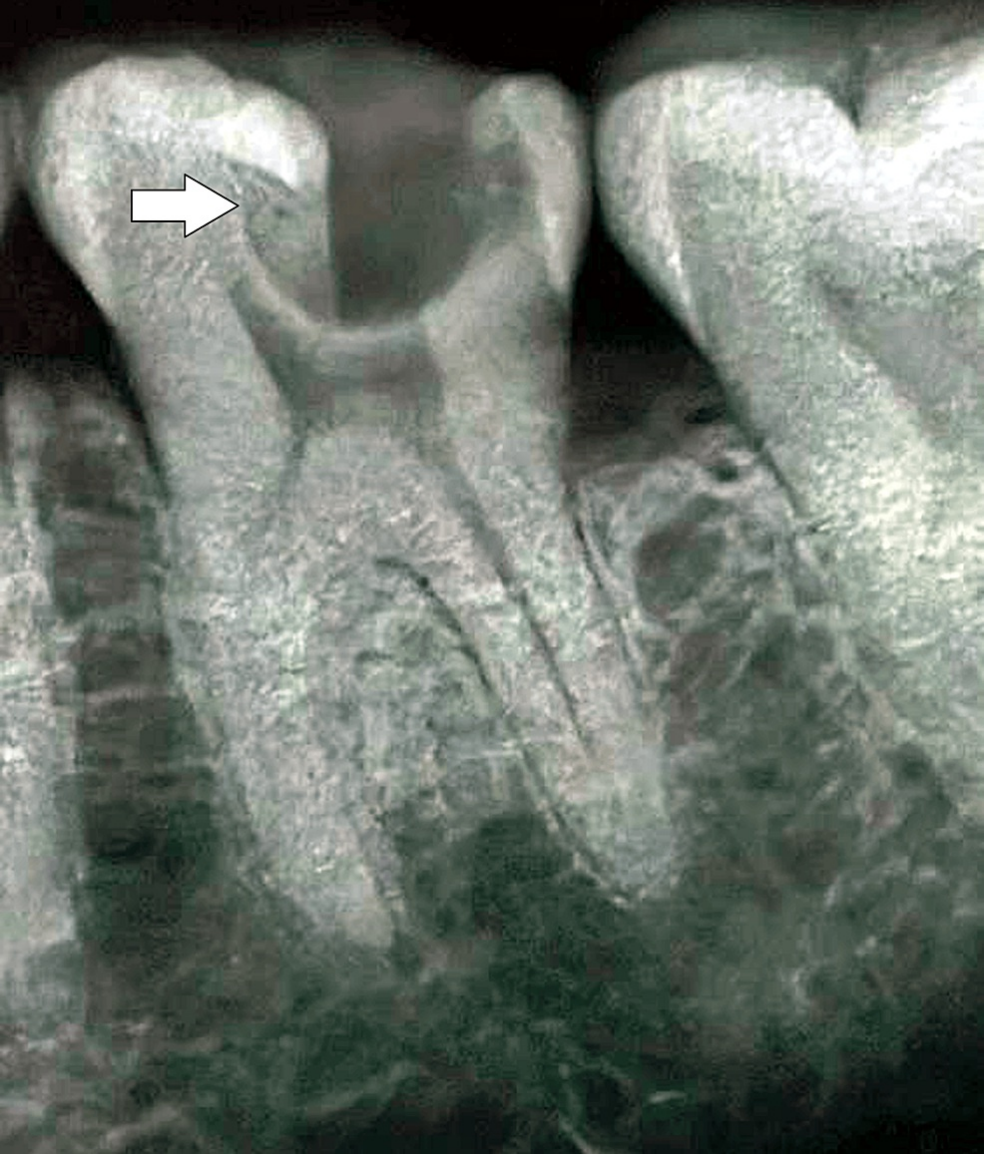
Diagnostic radiograph showing deep occlusal caries in tooth 46 Courtesy: Shreeya Panchal
